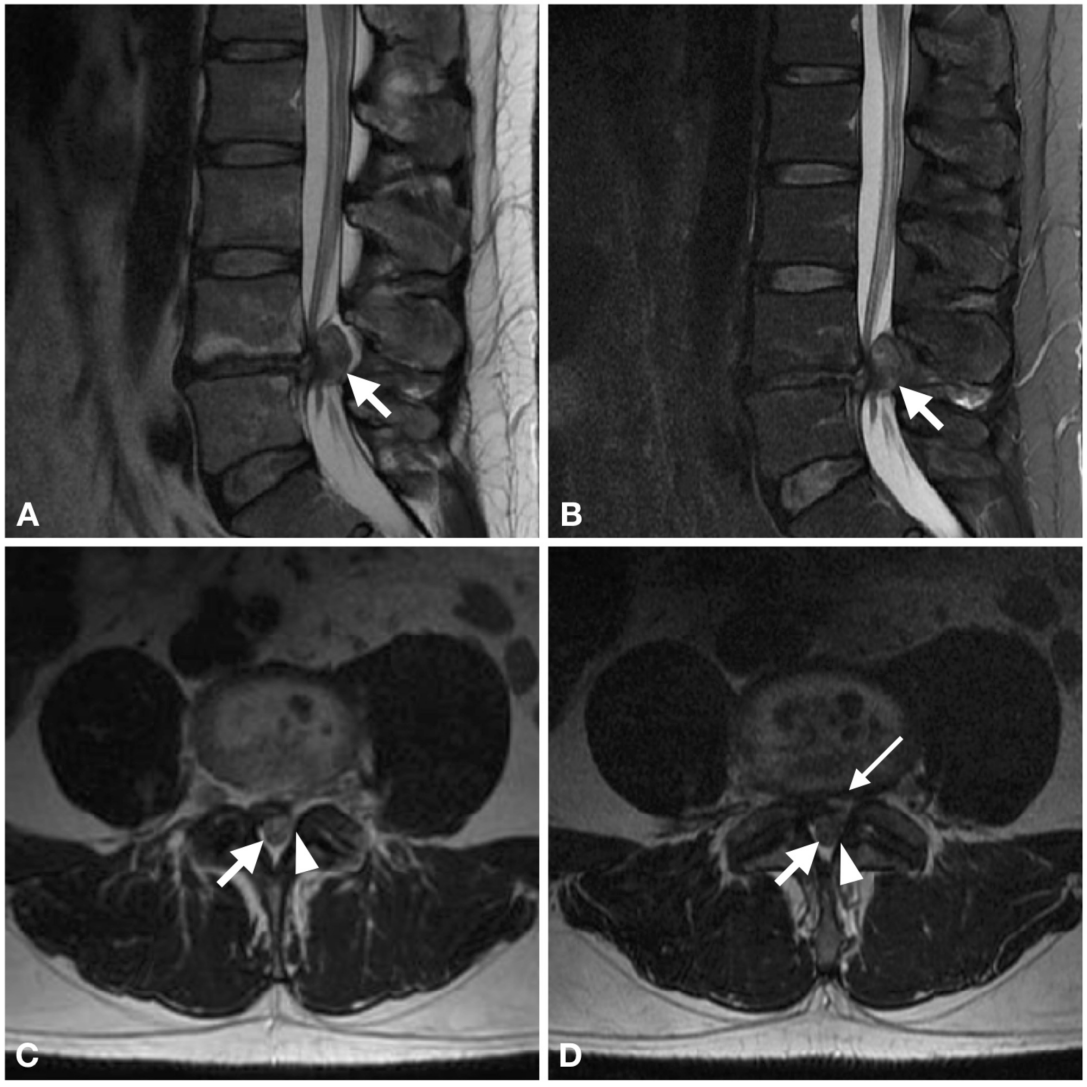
MRI of a 40-year-old male with acute onset of progressive bilateral leg weakness, back pain, and urinary retention Sagittal T2-weighted (A) and T2-weighted with fat suppression (B) images demonstrating a large T2-hyperintense mass (white arrow, A-D) in the L4-5 epidural space. Axial T2-weighted images at the level of the L4 vertebral body (C) and L4-5 disc (D) show the mass present in the left lateral and dorsal epidural spaces, displacing the thecal sac anteriorly and to the right. The mass abuts the left L4-5 ligamentum flavum (arrowhead, C-D), but does not create an obvious connection with the facet joint. It is, however, contiguous with the dorsal aspect of the disc space (skinny arrow, D). MRI: magnetic resonance imaging
